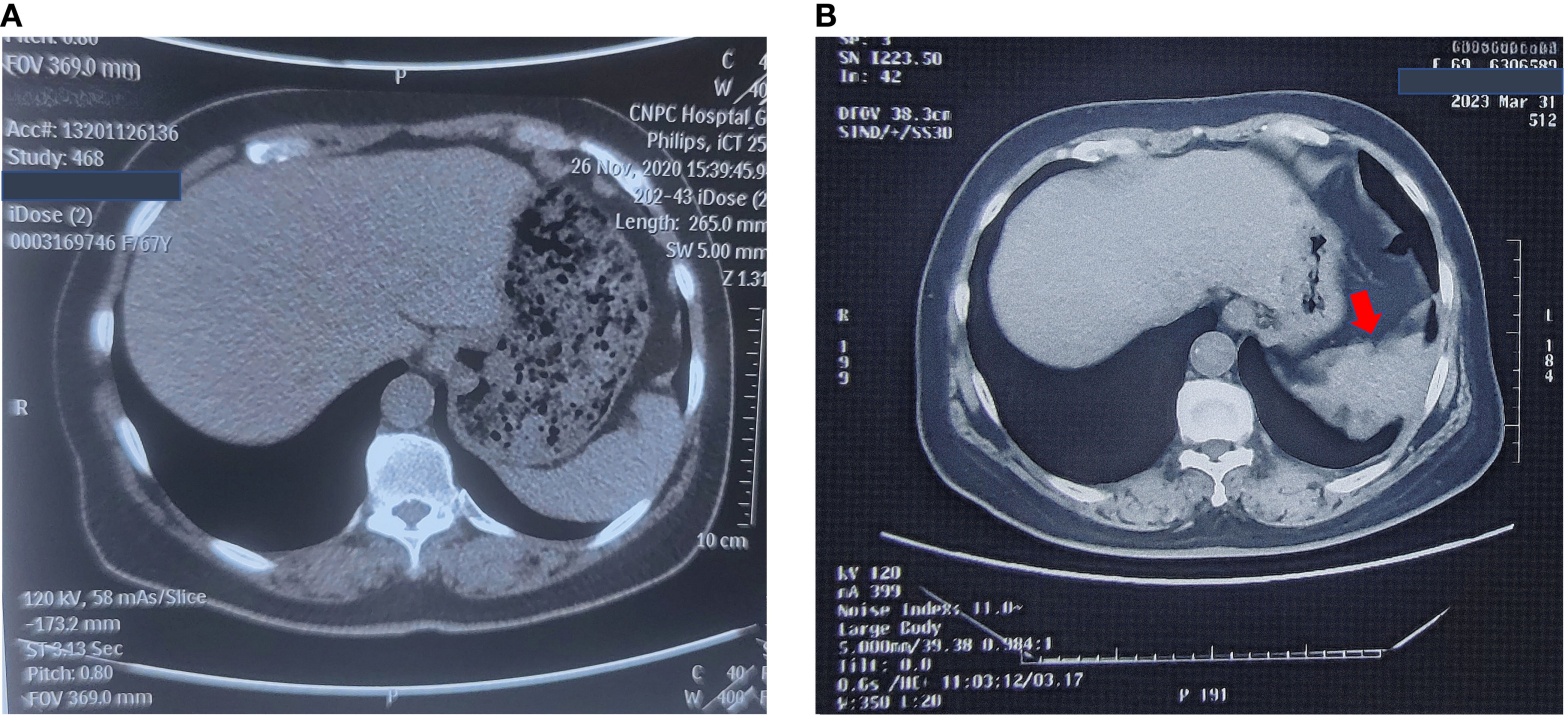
No-contrast abdomen CT changes before November, 2023. (A) No-contrast abdomen CT on November 26^th^, 2020. (B) No-contrast abdomen CT on March 31^st^, 2023.
